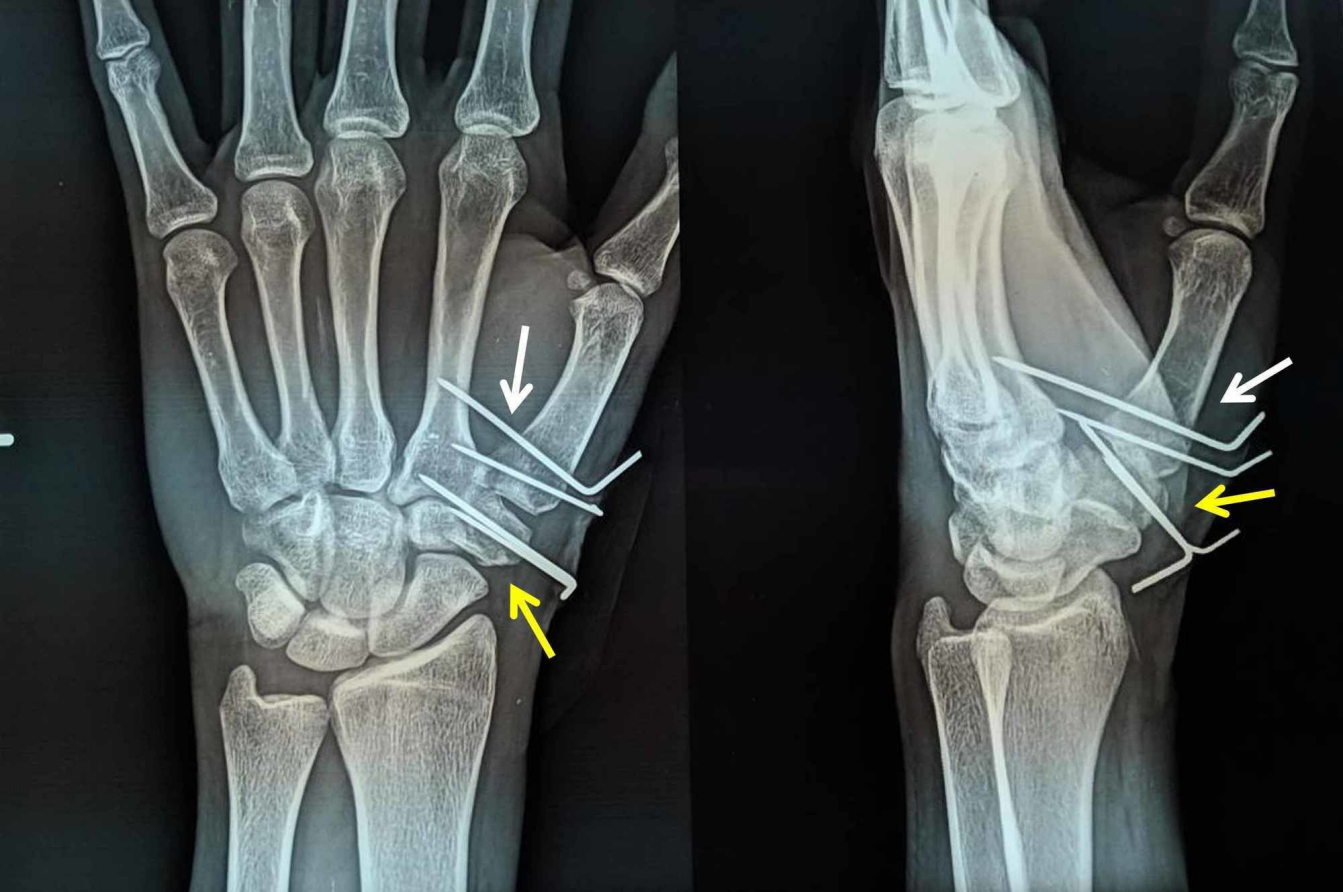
Anteroposterior and lateral radiographs of the hand at six weeks following surgery showing good fracture healing White arrows - Bennett fracture; yellow arrows - trapezium fracture
